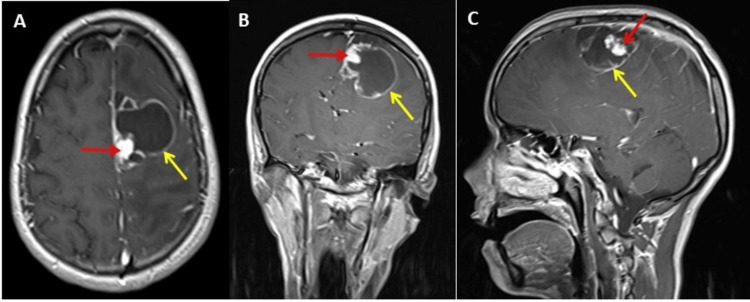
Post-contrast axial, coronal, and para-sagittal magnetic resonance imaging (MRI) of the brain. MRI brain post-contrast images axial (A), coronal (B), and para-sagittal (C); T1 images of the brain showing mural nodule abutting the pia matter and displaying avid enhancement (red arrows). Also, peripheral ring enhancement of the cystic lesion is evident (yellow arrows).
